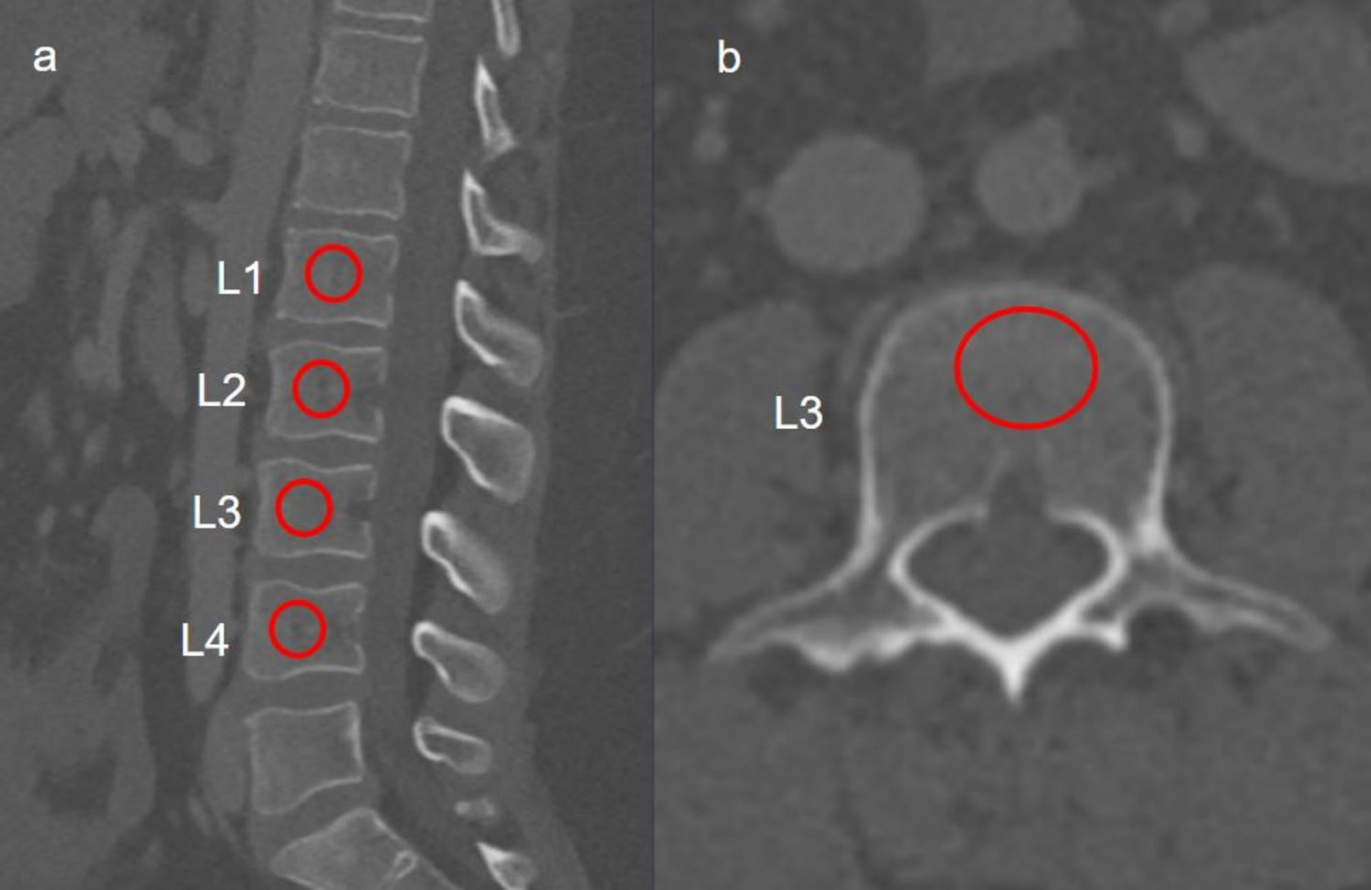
Sagittal (a) and axial (b) CT images of the lumbar vertebrae demonstrating the measurement of HU values using a circular region of interest (ROI; red circle). HU values were obtained from the trabecular portion of the vertebral body, avoiding cortical margins and vascular channels
